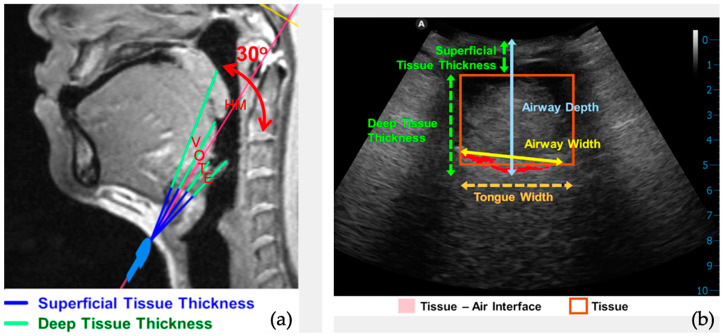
(a) The 30° segment of the upper airway; HM, hyoid-external meatus. (b) Transverse view of ultrasonographic images.
